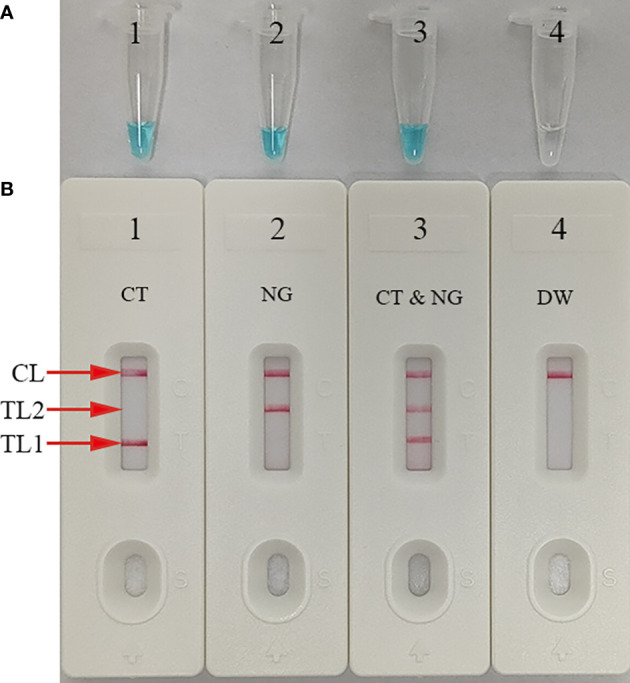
mLAMP product confirmation and verification The mLAMP products were simultaneously identified using (A) malachite green and (B) the AuNPs-LFB. Tube/AuNPs-LFB 1 shows a positive *C*. *trachomatis* result. Tube/AuNPs-LFB 2 shows a positive *N. gonorrhoeae* result. Tube/AuNPs-LFB 3 shows a positive *C*. *trachomatis* and *N. gonorrhoeae* result. Tube/AuNPs-LFB 4 shows negative control (DW). Key: CL, control line; TL1, test line one; TL2, test line two; CT, *C*. *trachomatis*; NG, *N. gonorrhoeae*.
